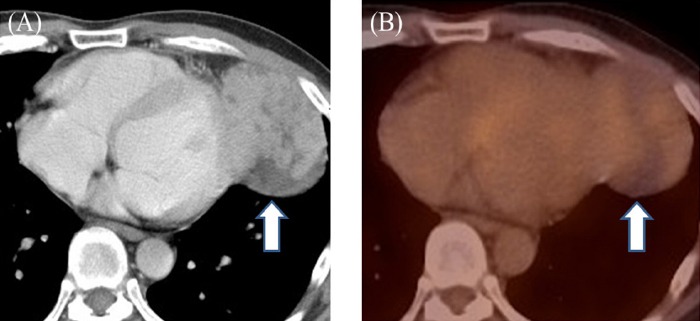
(A) Contrast-enhanced CT image shows a partially ill-defined, heterogeneous left-anterior mediastinal tumor (arrow); (B) PET/CT image shows low FDG uptake (arrow); SUV_max_, MTV and TLG estimated at 2.0, zero and zero, respectively A 44-year-old man with type B1 thymoma
